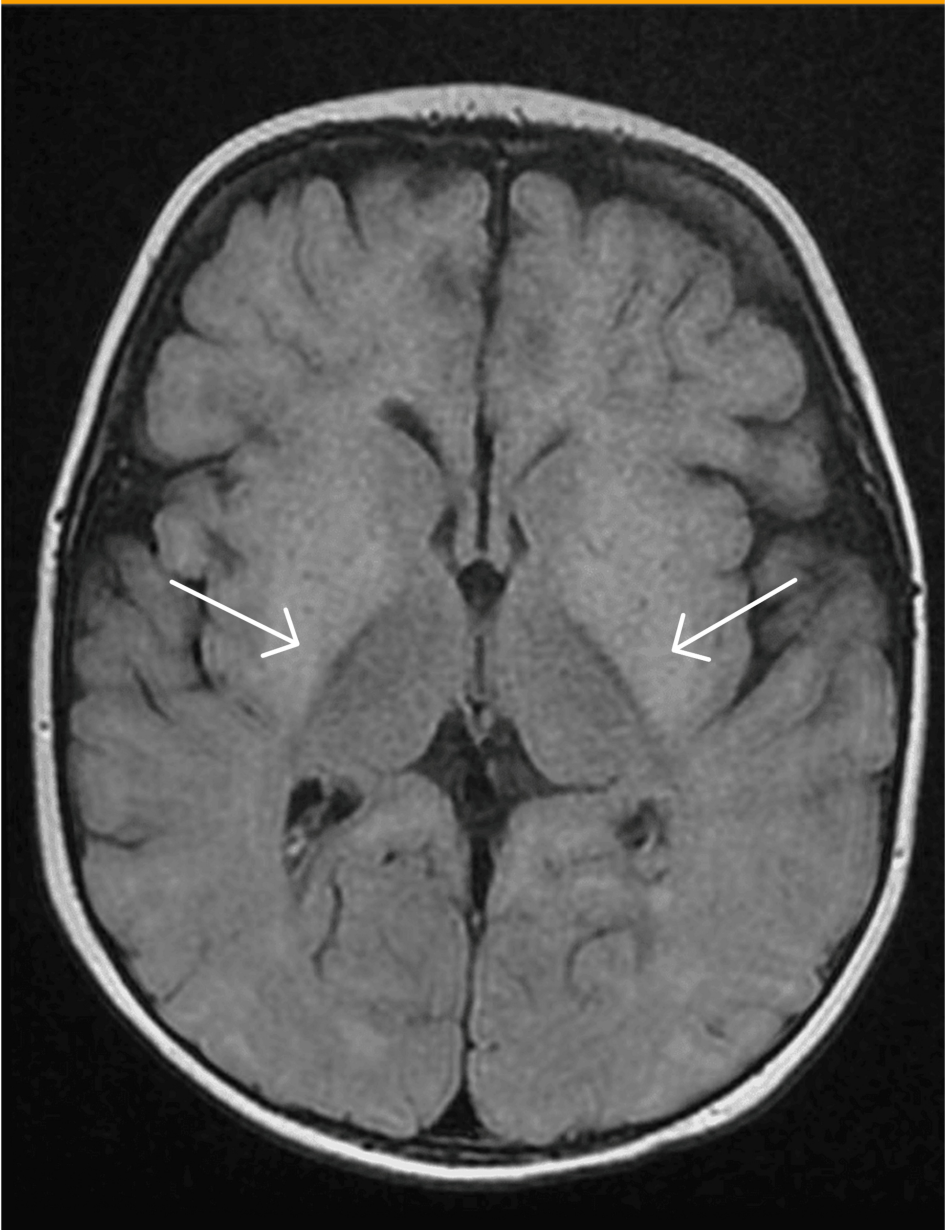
Fluid-attenuated inversion recovery MRI showing bilateral symmetric areas of diffusion restriction mainly involving the basal ganglia including the caudate nucleus putamen and globus pallidus pallidum.
